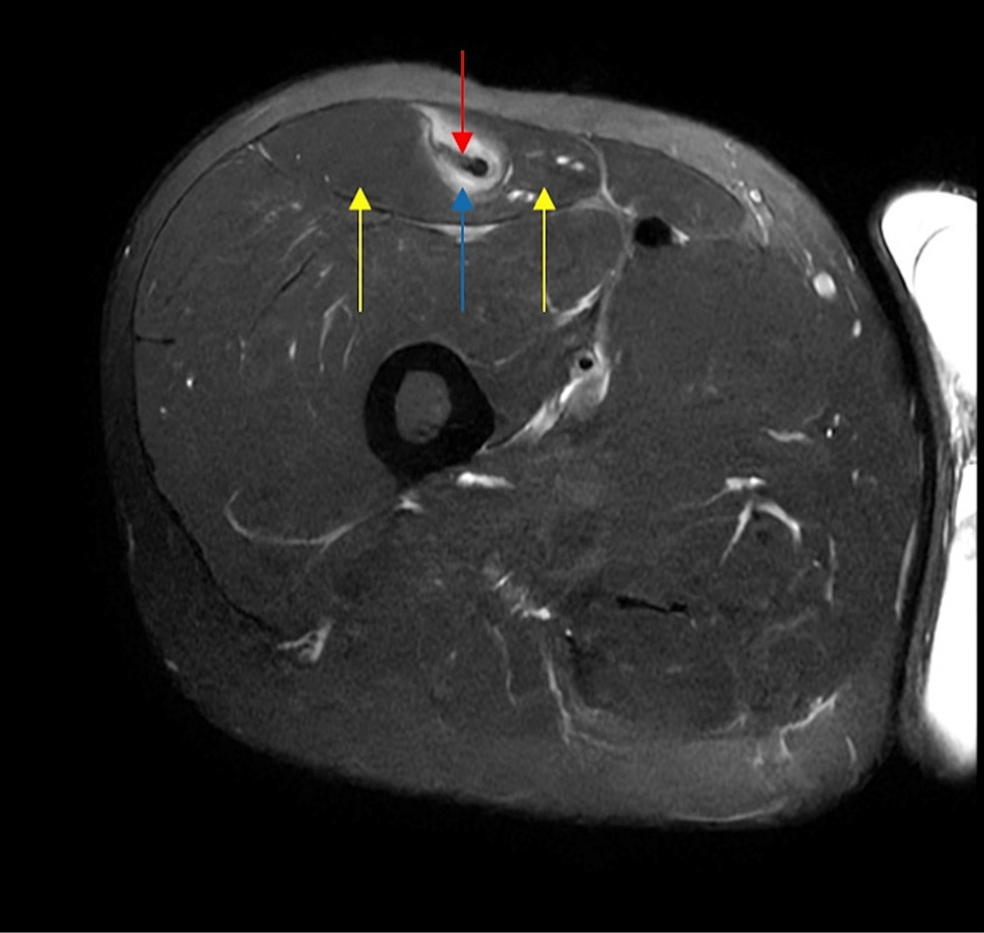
Axial T2 fat-suppressed MRI through the mid-thigh demonstrating an edematous and torn inner bipennate intramuscular portion (red arrow), the finger, with separation and a small amount of intervening fluid and likely blood products (blue arrow) from the surrounding outer unipennate portion of the muscle (yellow arrows), the glove, which appears normal. MRI, magnetic resonance imaging
